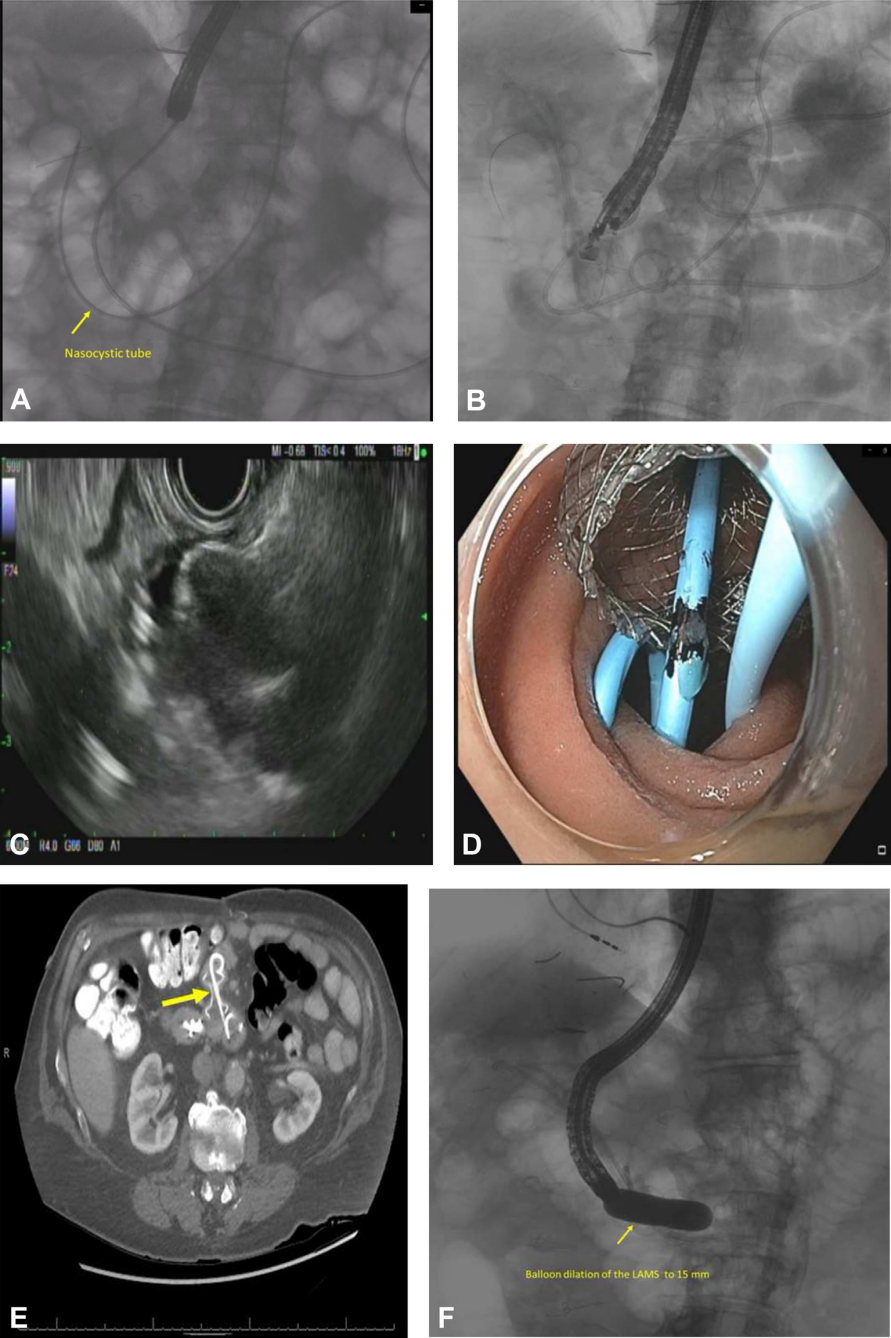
EUS-guided jejuno-jejunostomy with electrocautery-assisted 15- × 10-mm LAMS in a patient with total gastrectomy with Roux-en-Y esophagojejunostomy to facilitate cholangioscopy with electrohydraulic lithotripsy. A, A fluoroscopic image showing the orojejunal tube positioned to opacify the afferent limb. B, Fluoroscopic image indicating the EUS scope positioned in the roux limb. C, Under EUS guidance, the distal flange of the LAMS is deployed followed by the proximal flange using a free hand method. D, Endoscopic view of the deployed LAMS with a plastic stent traversing it. E, A coronal CT image confirming successful creation of the jejuno-jejunostomy. F, Balloon dilation of the LAMS to 15 mm 4 weeks after deployment. *LAMS*, Lumen-apposing metal stent.
